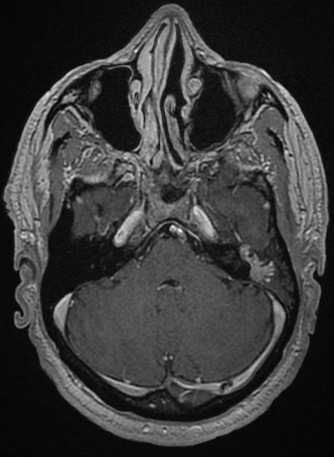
T1 MRI post-contrast demonstrating mild enhancement of the lesion in the left middle ear
